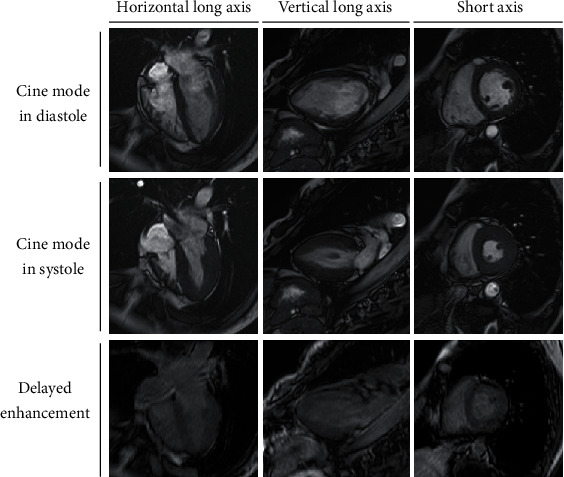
Cardiac magnetic resonance imaging showing no findings of morphological abnormalities or delayed enhancement.
